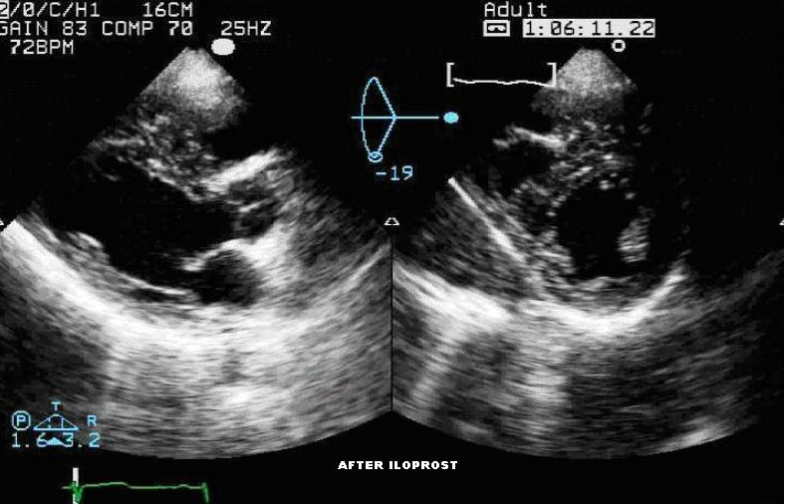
Transthoracic echocardiogram showing left ventricle long and short axis views after iloprost nebulization. In the echocardiographic evaluation after iloprost administration we can detect a reduction in right ventricular dimension, with a proportional increase in left ventricular dimension and performance. The correction of the septal shift secondary to right ventricular overload also contributes to a smaller right ventricle and expanded left ventricle.
